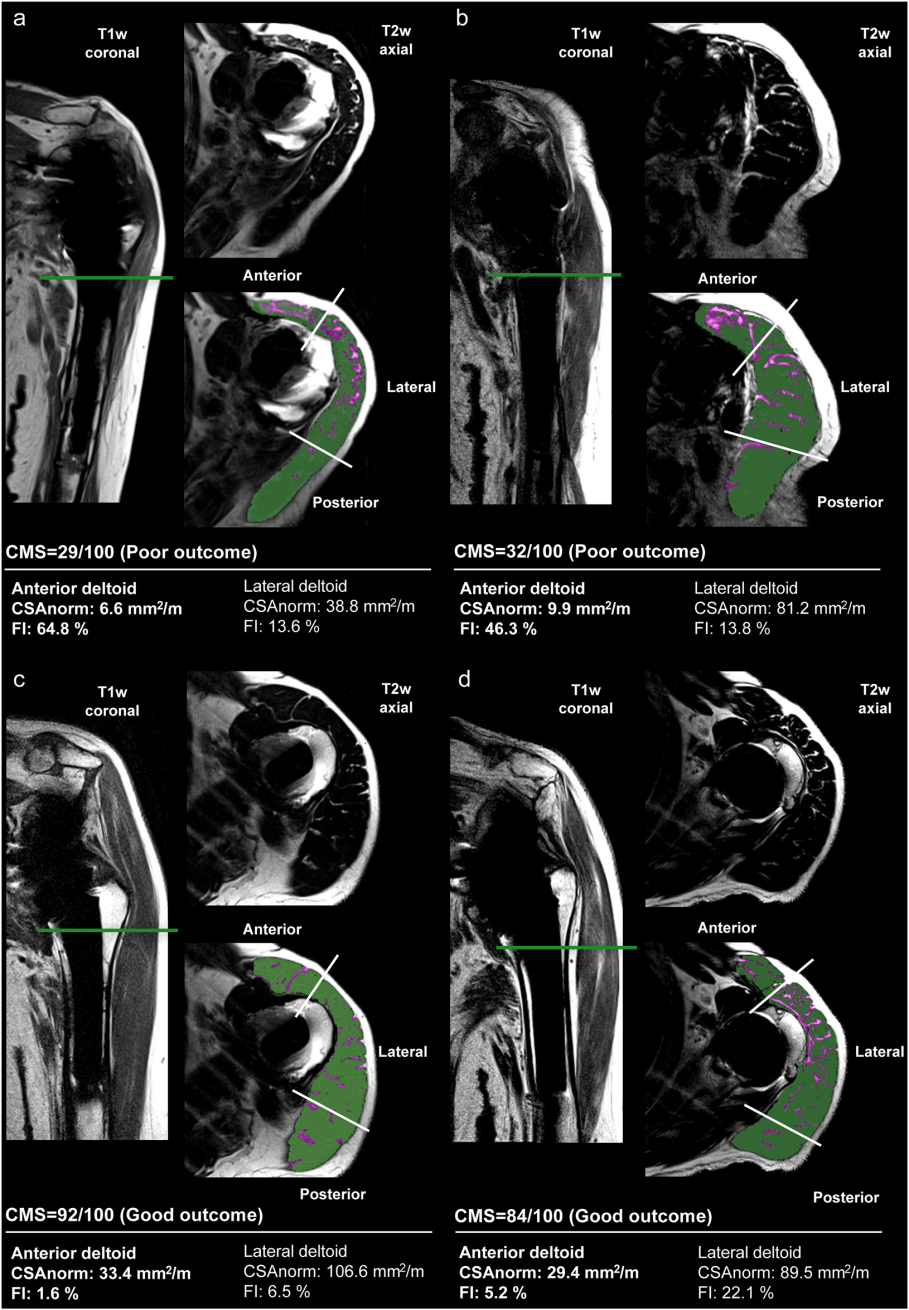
Representative cases of poor and good clinical outcomes after RTSA are demonstrated by T1w coronal and T2w axial MRI sequences with WARP metal artifact reduction and corresponding segmentation overlay. a, b Poor outcome cases exhibit markedly higher anterior deltoid FI compared to (c, d) good outcome cases. Note that good functional outcomes can occur despite moderate lateral deltoid FI. MRI-derived deltoid muscle parameters for the anterior and lateral deltoid are provided below each case. In all cases, the left shoulder is featured. CSAnorm, cross-sectional area normalized to patient height; FI, fatty infiltration; CMS, constant-Murley score; WARP, Siemens sequence for metal artifact reduction, including VAT
